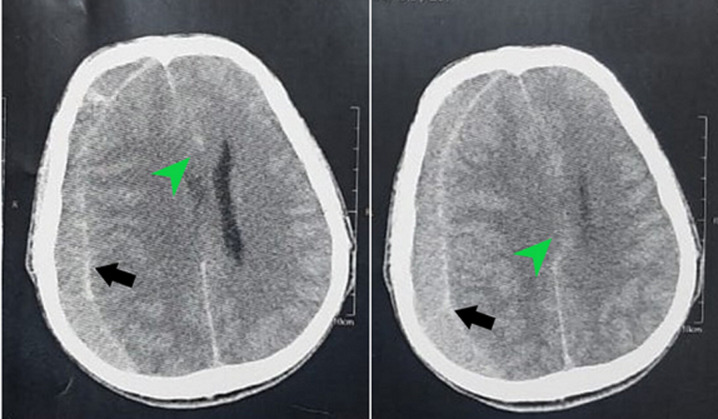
preoperative CT scan of the head in axial planes
